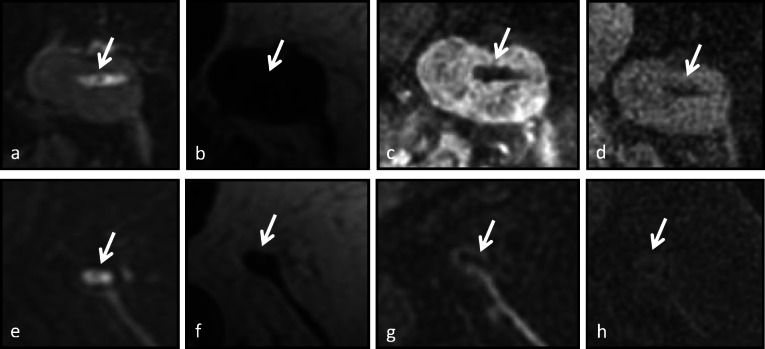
A 59-year-old female with newly diagnosed left breast invasive ductal carcinoma grade 3 with known metastatic left axillary lymphadenopathy. a–d Initial staging magnetic resonance imaging (MRI) performed 21 days after HydroMARK placement. The marker (arrow) is seen with high confidence on the T2-weigheted water-only fast spin-echo image (a), the unenhanced fat-suppressed three-dimensional T1-weighted spoiled gradient-recalled image (c), and the first contrast-enhanced fat-suppressed three-dimensional T1-weighted spoiled gradient-recalled image (d), while it is seen with low confidence on the T2-weigheted fat-only fast spin-echo image (b). e–h NAT follow-up MRI performed approximately 6 months after marker placement shows decreased size of lymph node. After NAT, the marker (arrow) is seen with high confidence on the T2-weigheted water-only fast spin-echo image (e) and the first contrast-enhanced fat-suppressed three-dimensional T1-weighted spoiled gradient-recalled image (h) sequences, while it is seen with low confidence visibility on the T2-weigheted fat-only fast spin-echo image (f) and the unenhanced fat-suppressed three-dimensional T1-weighted spoiled gradient-recalled image (g). Note, the decreased T2 brightness of the marker gel content 6 months after placement (e) compared to the initial MRI after placement (a)
